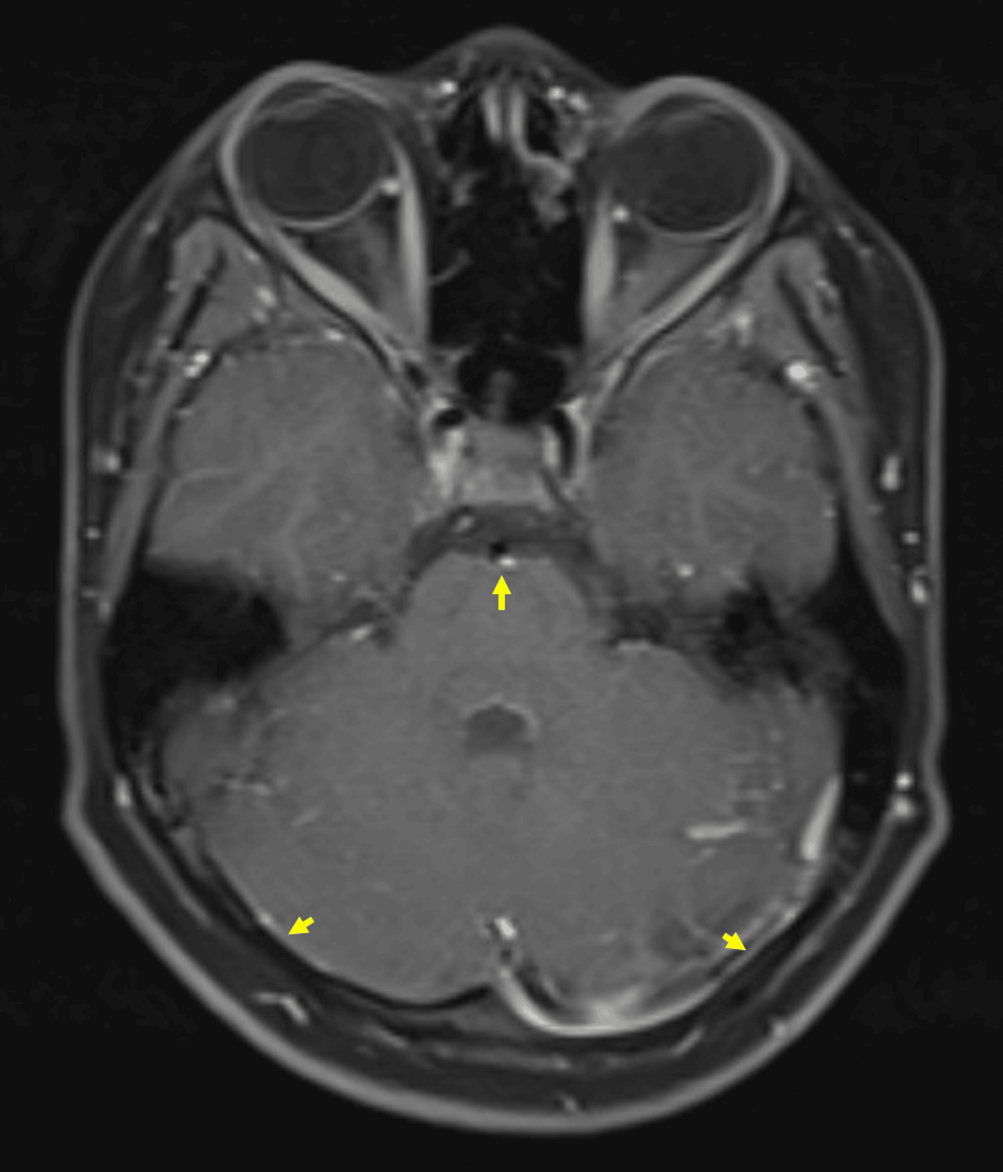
Axial T1-weighted post-contrast brain MRI Diffuse basal leptomeningeal enhancement (arrows), predominantly involving the prepontine and posterior fossa cisterns, consistent with acute bacterial meningitis.
